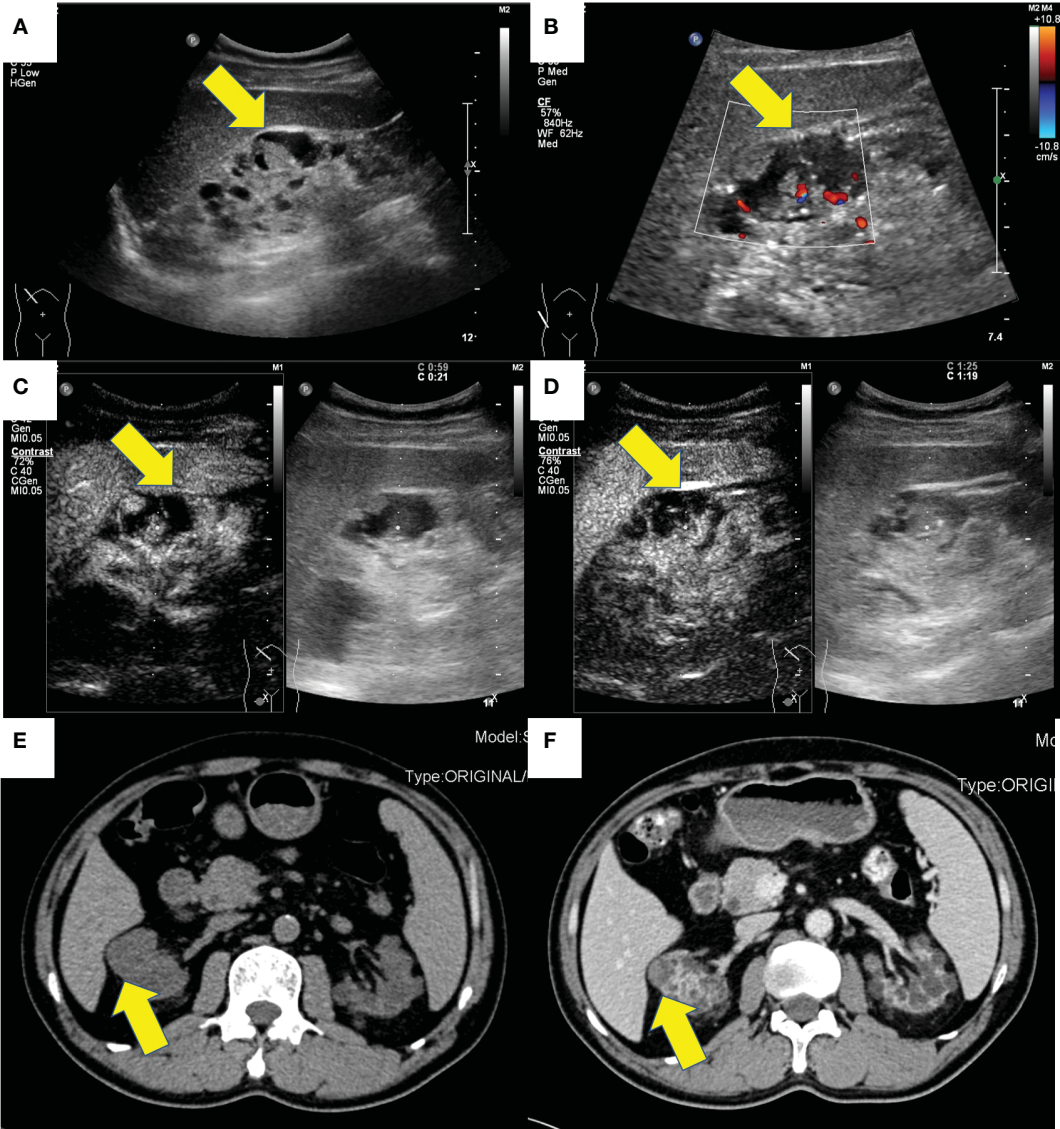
The ultrasound and CT images of a 58-year-old male (case 1). (A) Gray-scale, (B) color Doppler, (C) cortical phase and (D) medullary phase sonograms of the mass in right kidney. (E) non-enhanced CT and (F) contrast-enhanced CT of the mass of right kidney.
